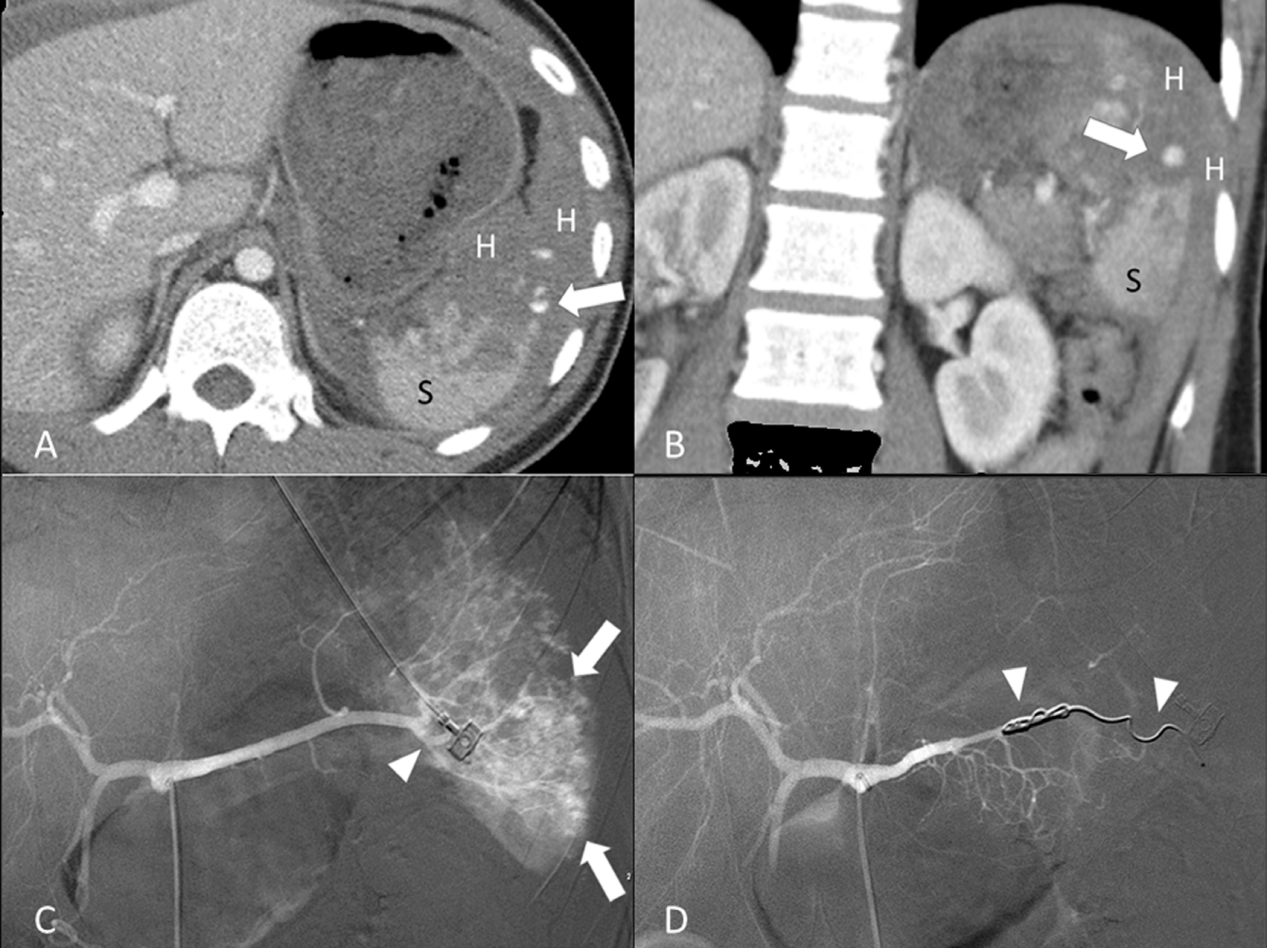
19-year-old male of grade IV splenic injury underwent combined transcatheter arterial embolization (A, B) Contrast-enhanced axial and coronal CT at portal venous phase shows a pseudoaneurysm (arrow) at the lacerated spleen (S) surrounded by hemoperitoneum (H). (C) Angiography discloses multiple tiny pseudoaneurysms (arrow) and the supplying branch artery (arrowhead). (D) Angiography after a successful combined embolization shows deployment of metallic coils (arrowheads) at the branch artery and main splenic artery distal to the dorsal pancreatic artery.
